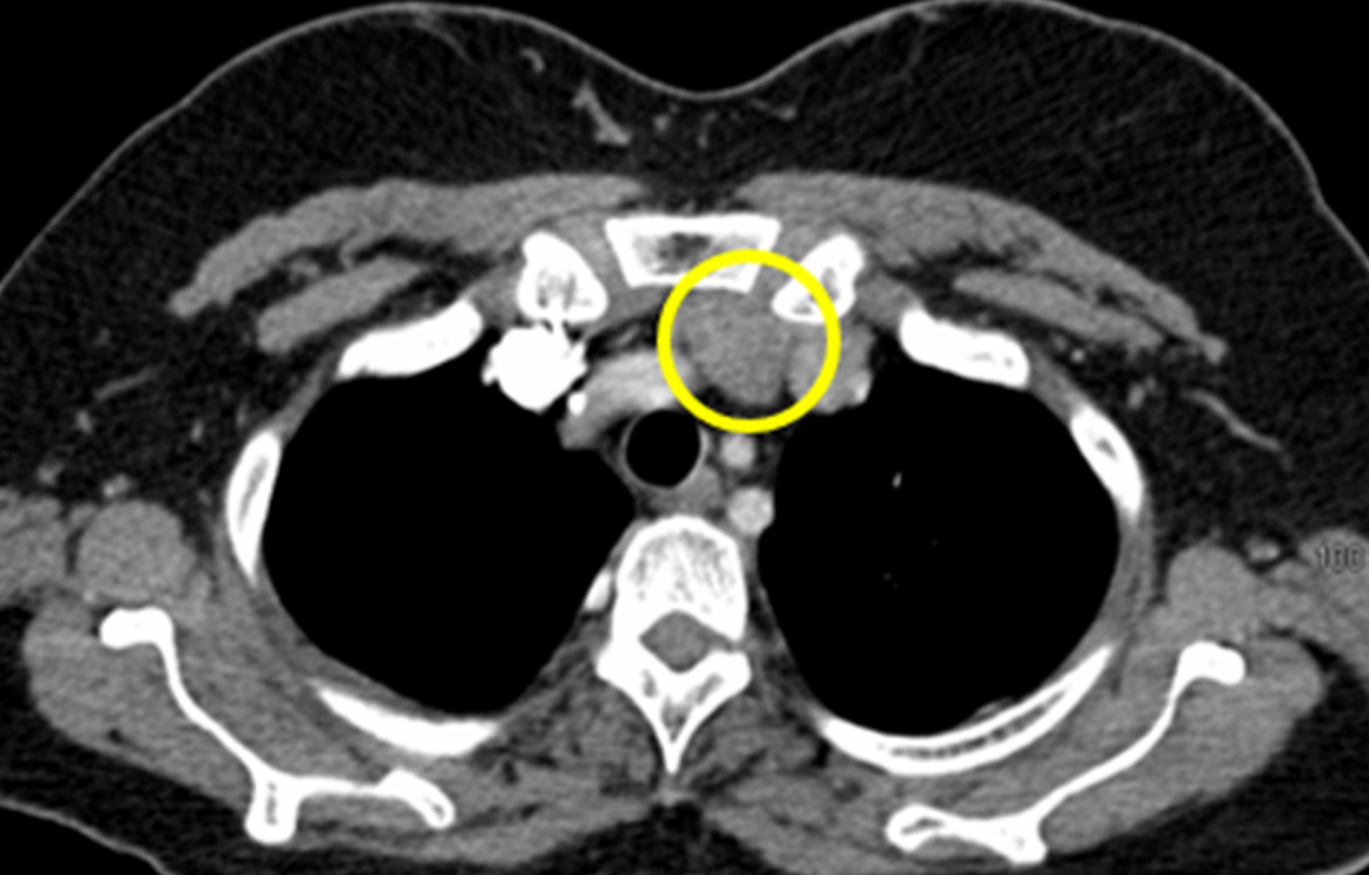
Axial Computed Tomography of the Chest Revealing an Anterior Mediastinal Mass The figure shows an axial computed tomography of the chest, revealing an incidental anterior mediastinal mass (yellow circle).
